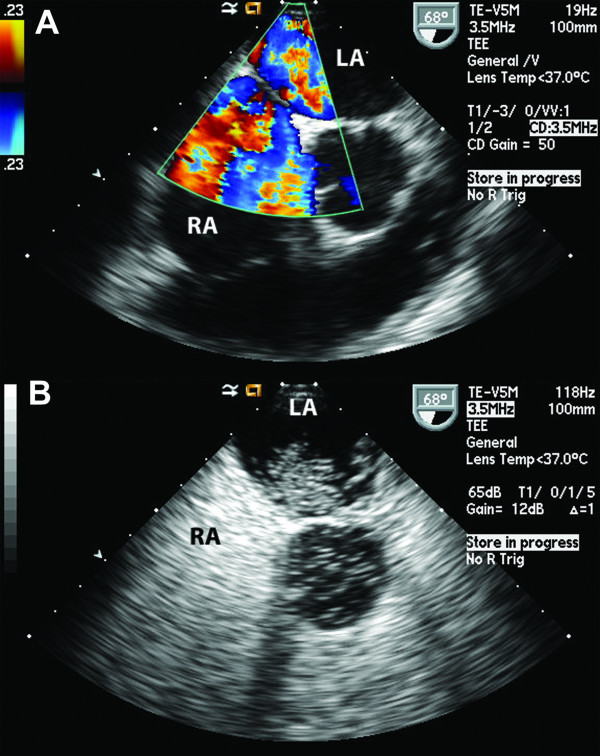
Transesophageal echocardiography during the patient's initial hospitalization. (A) A bidirectional interatrial shunt seen through the patent foramen ovale. RA, right atrium; LA, left atrium. (B) Injection of agitated saline through an upper extremity peripheral vein showing the appearance of bubbles crossing from the right to the left atrium. RA, right atrium; LA, left atrium.
